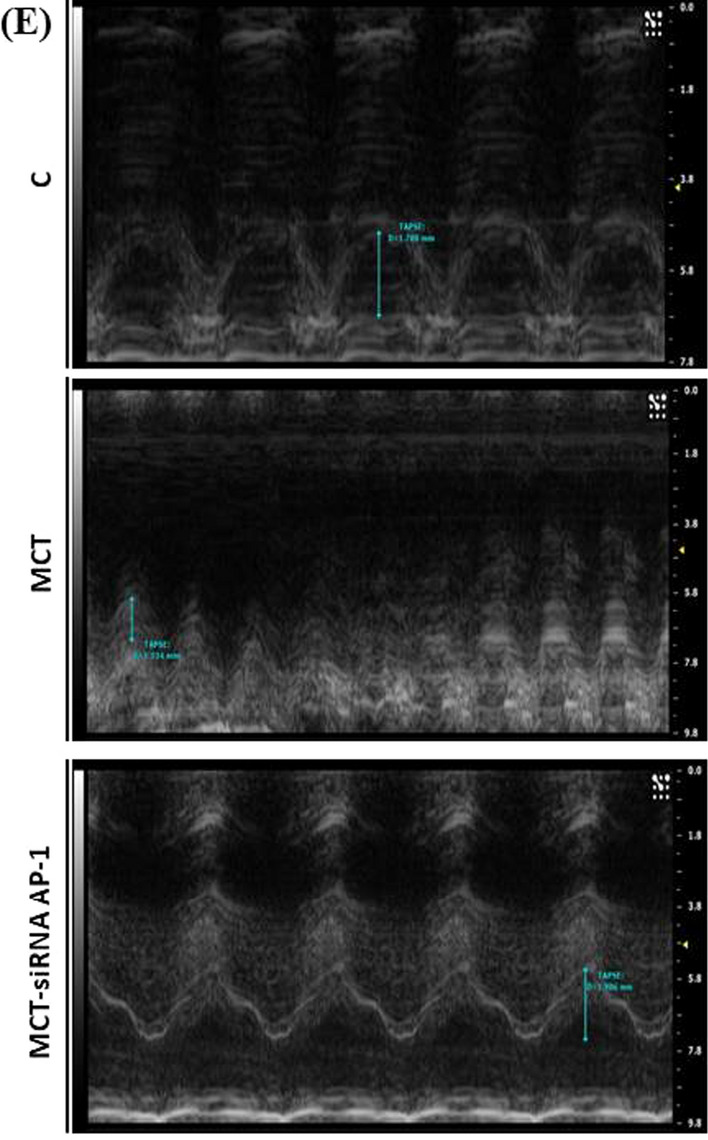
Changes in the blood flow, structure and function of pulmonary artery and right ventricle for the investigated experimental groups: C, MCT, MCT-siRNA AP-1. A Representative recordings obtained in pulsed wave Doppler-mode, which highlight the Velocity Time Integral (VTI, mm) and Doppler Peak Velocity (Vel, mm/s) of the pulmonary artery; B representative B-mode recordings, which highlight the inner diameter (mm) of the pulmonary artery; C Representative B-mode recordings, which highlight the area of the right and left ventricle in diastole (mm^2^); D Representative M-mode recordings, which highlight right ventricle wall thickness in diastole (RVWTd, mm); Representative M-mode recordings, which highlight proximal ventricle outflow chamber during systole and diastole (PRVOFd, PRVOFs, mm); E Representative M-mode recordings which highlight tricuspid annular plane systolic excursion (TAPSE, mm)
